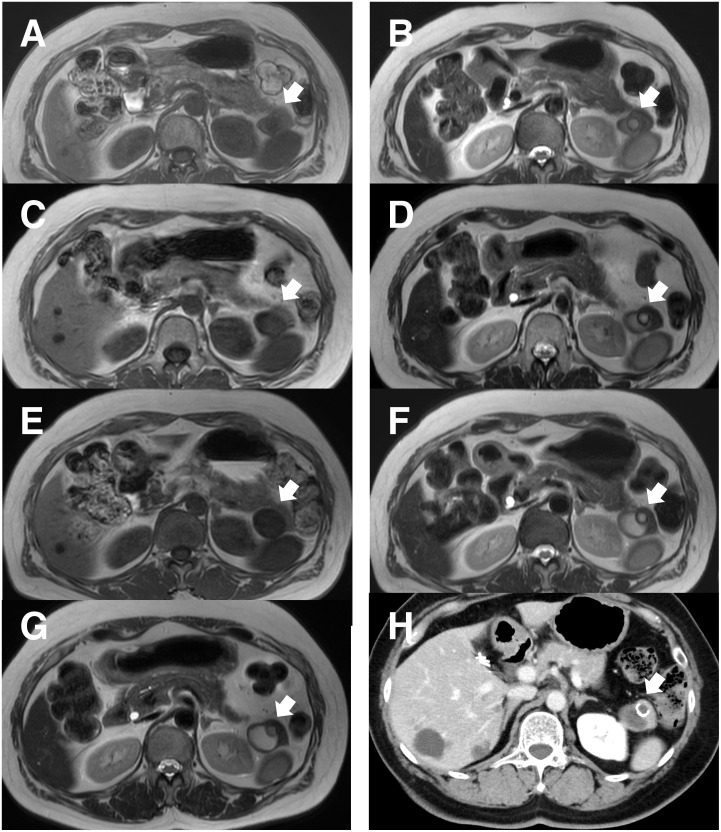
Serial image findings. (A, B) Initially, the tumor was a 13-mm cystic tumor with hypointense T1 (left panel) and slightly hyperintense T2 signals (right panel), displaying a “cyst-in-cyst” appearance (arrow). (C–F) Annually, the size gradually increased in both T1 (left panel) and T2 (right panel) images (arrow). (G) Finally, the size became 30 mm in diameter on T2 image, while the morphology remained unchanged (arrow). (H) CECT image confirmed a thick capsule with septal calcifications (arrow) but no solid components. CECT, contrast-enhanced CT
